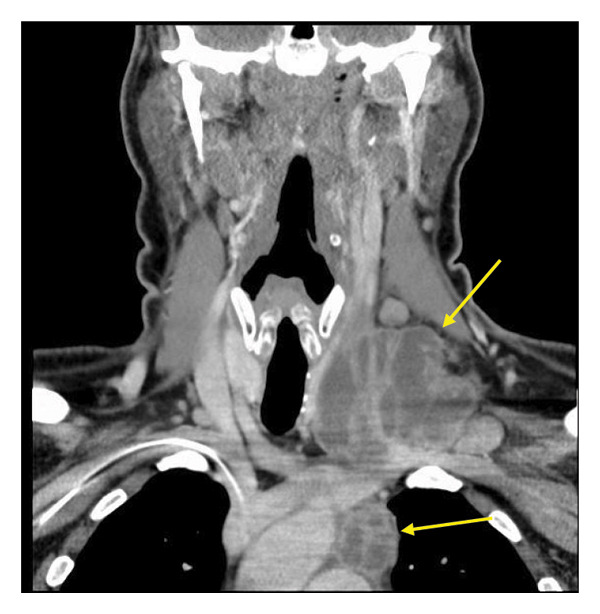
Coronal CT image of the large left‐sided neck abscess extending into the superior mediastinum.
